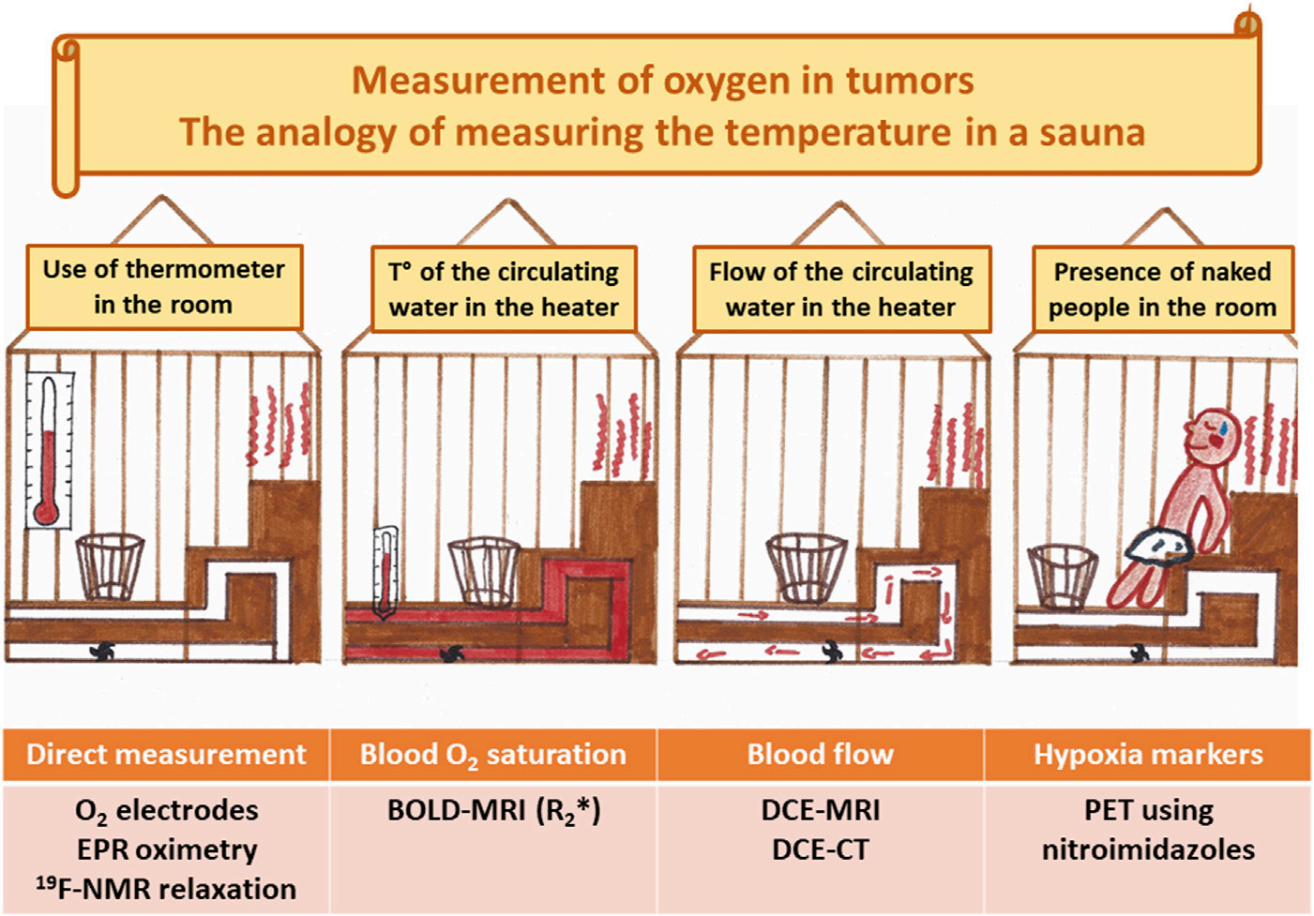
Comparison between measuring tumor oxygenation with hypoxia biomarkers and measuring temperature in a sauna. The direct way to measure the temperature is to use a thermometer. Translated in the hypoxia world, it corresponds to direct measurements of tissue oxygenation (pO_2_ histography, optic fibres, EPR oximetry, ^19^F-relaxometry). Another approach is to measure the temperature of the circulating water in the heater. Caution: we do not know if the door is open. This approach is analog to the measurements done using the oxygen blood saturation (R_2_* or BOLD-MRI). It provides information on the oxygen delivery through the blood. Another approach is to measure the flow of the circulating water in the heater. Caution: we do not know if the door is open and if the circulating water is hot. It is comparable with DCE-MRI. The method only considers the delivery, no information is provided regarding the oxygenation. Finally, we may look for the presence of naked (or almost) people in the room. While we do not know the temperature, their presence likely means a hot temperature inside the sauna. This situation is analog to the accumulation of nitroimidazoles inside hypoxic cells. We do not know the real oxygenation of the tissue, but it is likely hypoxic.
